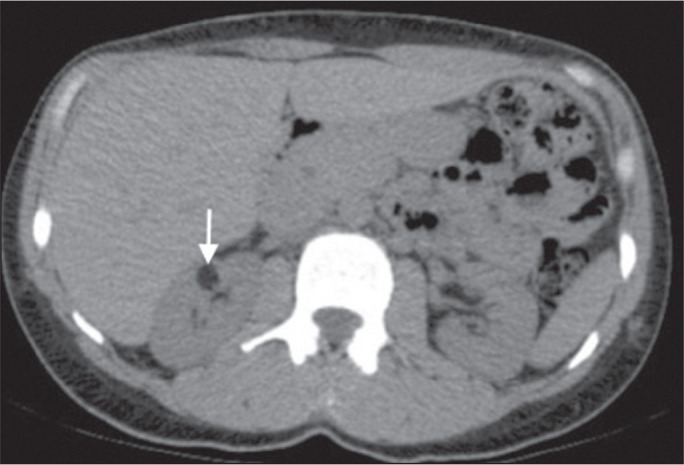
A 39-year-old female with dyspnea. Chest CT showing a very
